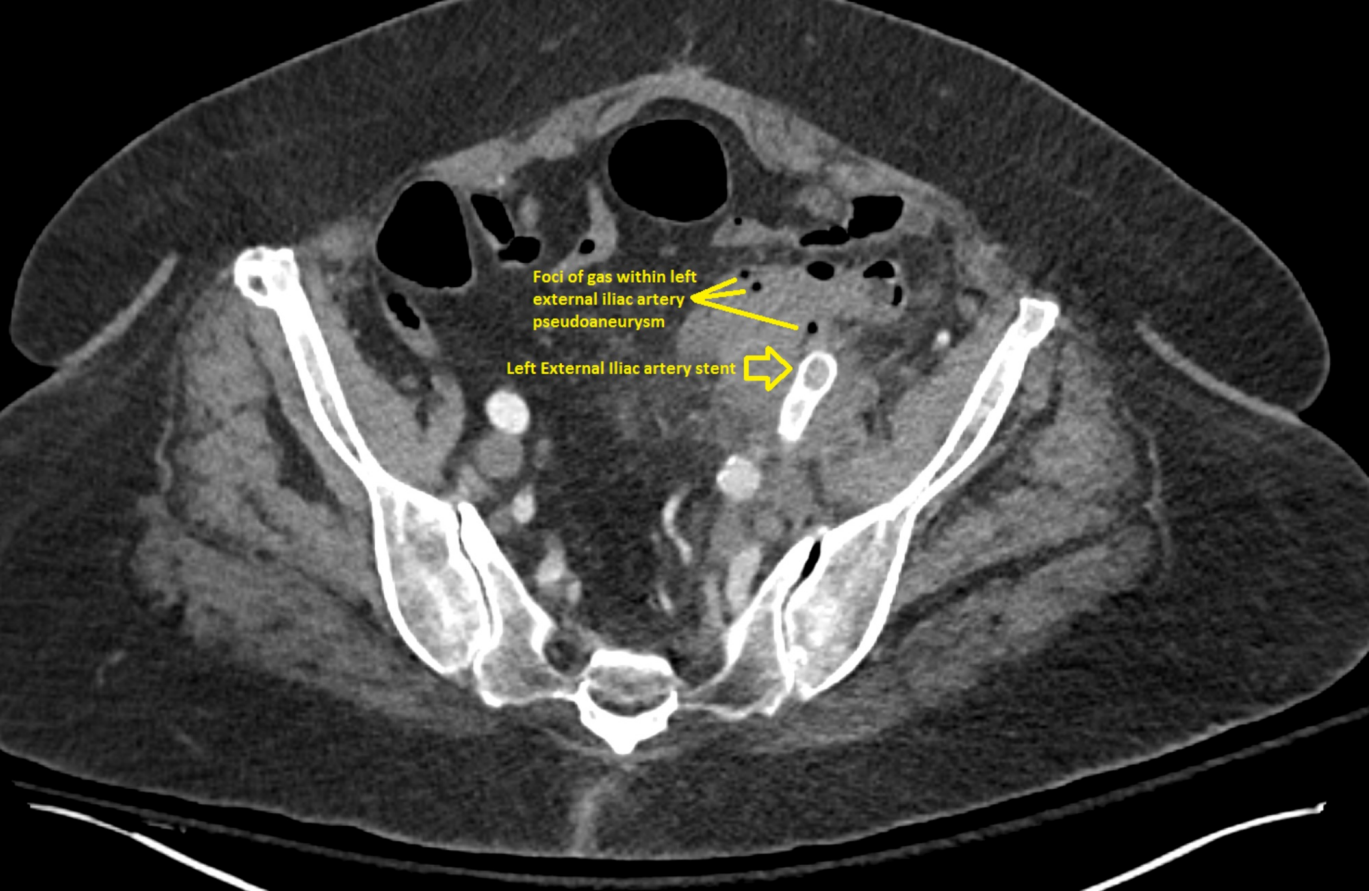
Computed tomography (CT) angiogram of the abdomen and pelvis showing patent left external iliac artery stent with foci of gas within the left external iliac artery pseudoaneurysm
